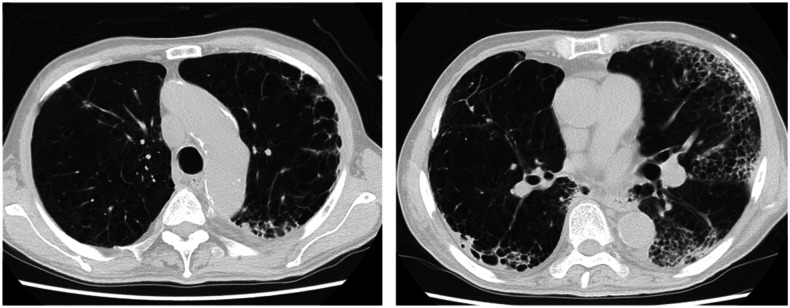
Computed tomography (CT) of the lung. Chest CT revealed diffuse low attenuation areas and increased concentrations along the circumference of the emphysema in a peripheral, bilateral, posterior, and lower lung zone distribution.
